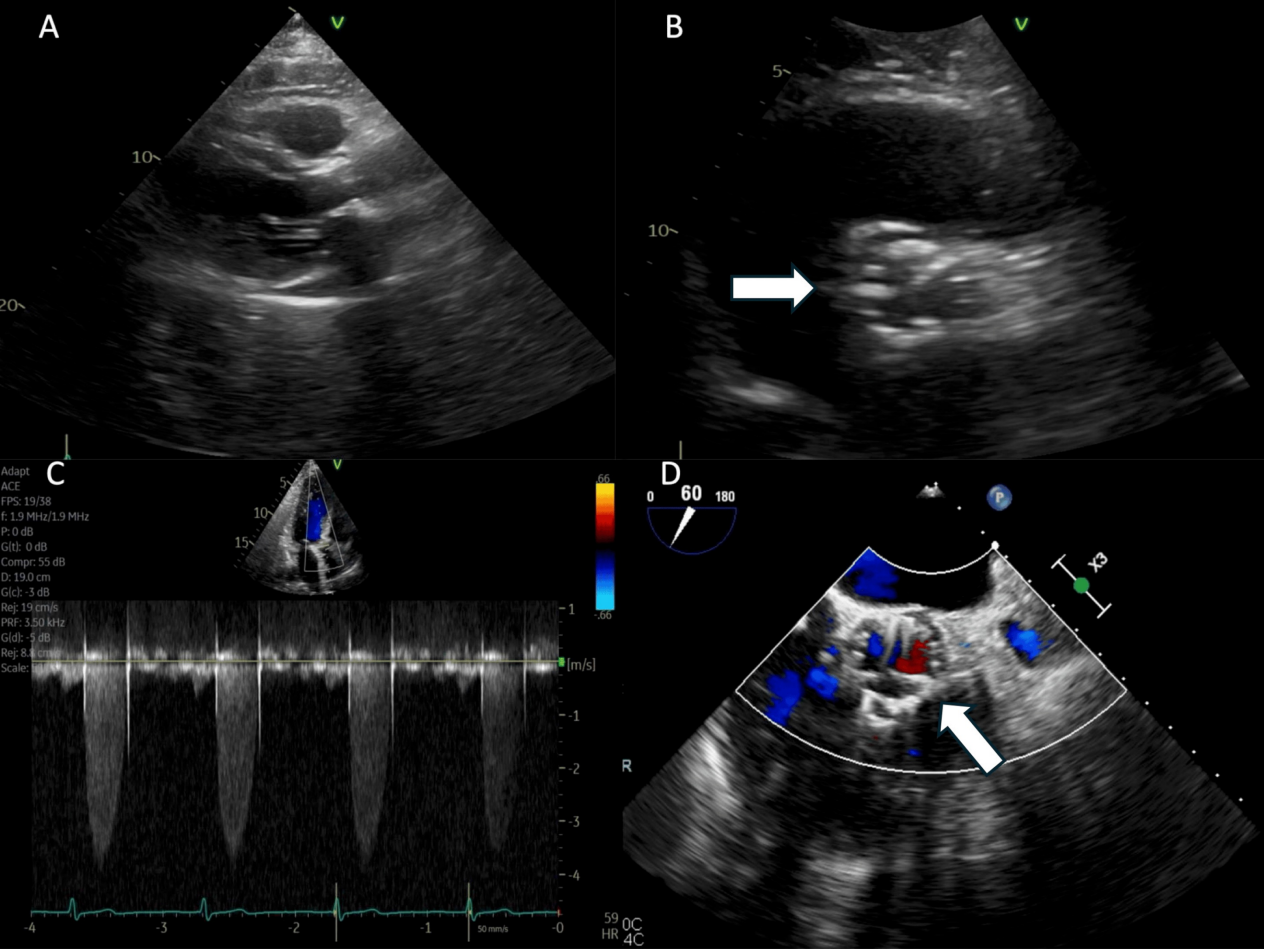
Preoperative transthoracic echocardiogram Normal left ventricular ejection fraction, >55%. 1B, 1C: Restricted leaflet motion (white arrow), mean pressure gradient 34 mmHg, aortic valve area 0.9 cm^2^, 1D: No significant aortic insufficiency (white arrow)
